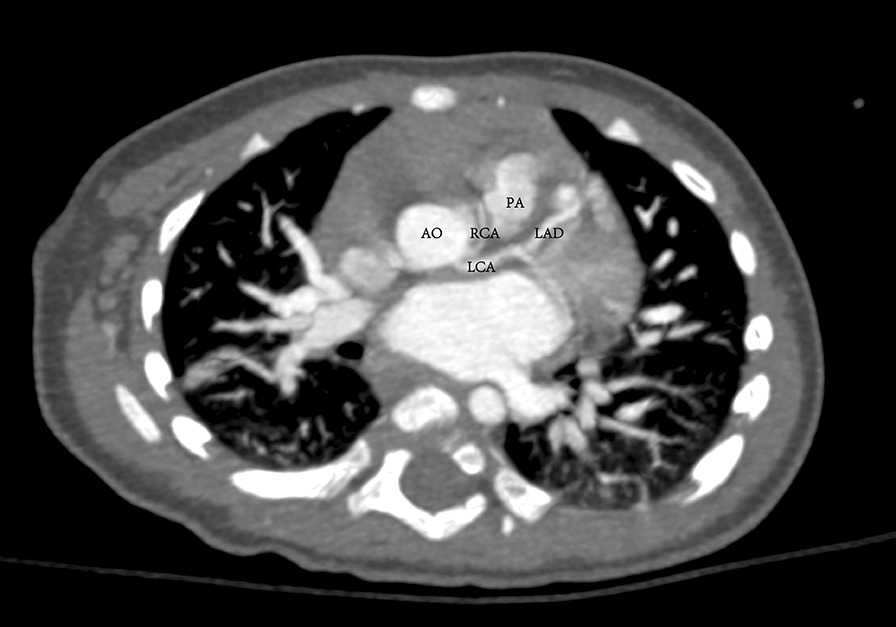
Computed tomography (CT) revealed both anomalous RCA and the normal LCA arose from the left coronary sinus, which coursed between the aorta and the pulmonary artery without an intramural segment
